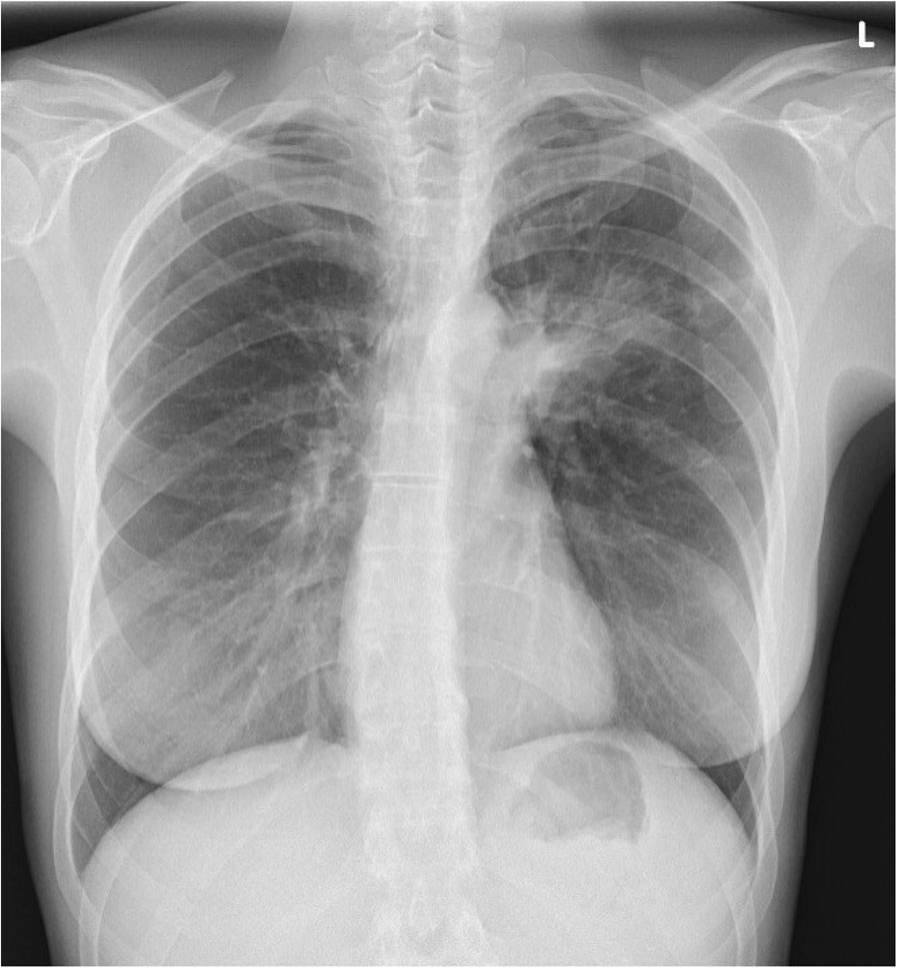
Chest X-ray showing a consolidation in the left hilum and left upper lobe
